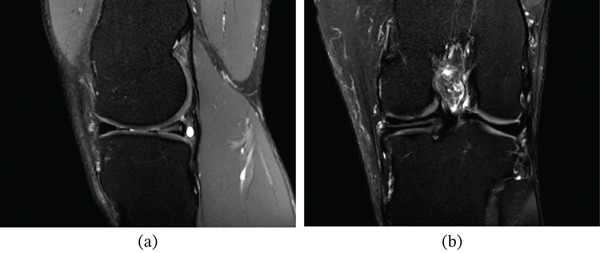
Sagittal (a) and coronal (b) T2‐weighted MRI images demonstrating a horizontal cleavage tear through the posterior horn of the medial meniscus.
